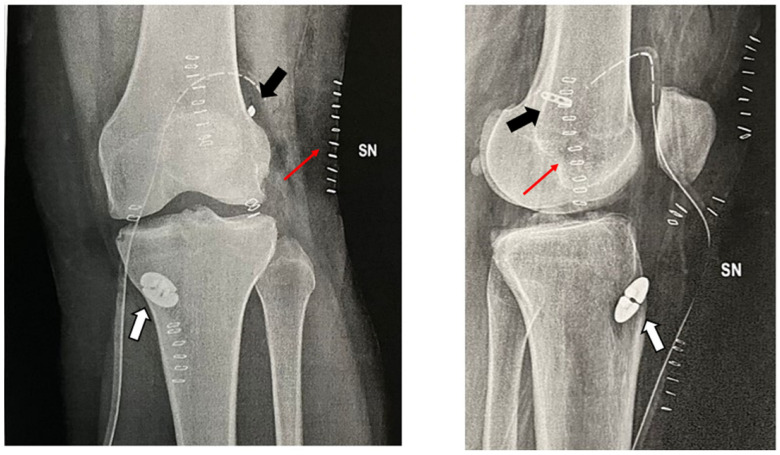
Post-operative X-rays. The surgical drainage is still in place, and the graft is fixed with two adjustable loop suspensory devices (black arrow) and a dedicated button on the tibial side (white arrow). On the lateral projection, only the skin staples (thin red arrow) can be appreciated at the level of the ITB harvesting site, since no additional hardware was used to fix the ITB strip.
